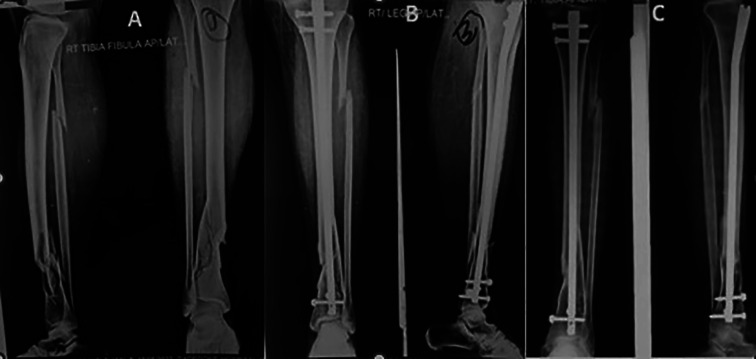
(A) Comminuted fracture distal tibia, (B) post op x-ray with suprapatellar nail and (C) six months follow up. Union of fracture.
